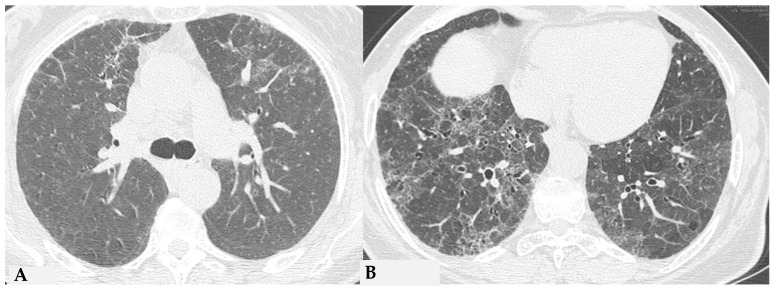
Truly indeterminate HP pattern. Chest HRCT, axial scan HRCT pattern characterized by mild fibrotic changes of a limited extent with patchy GGO in the upper lobes (A); the lower lobes show GGO, fine reticulations with modest mosaic attenuation and rare traction bronchiectasis/bronchiolectasis (B) with a “truly indeterminate” pattern according to new HP guidelines (2020) [4].
